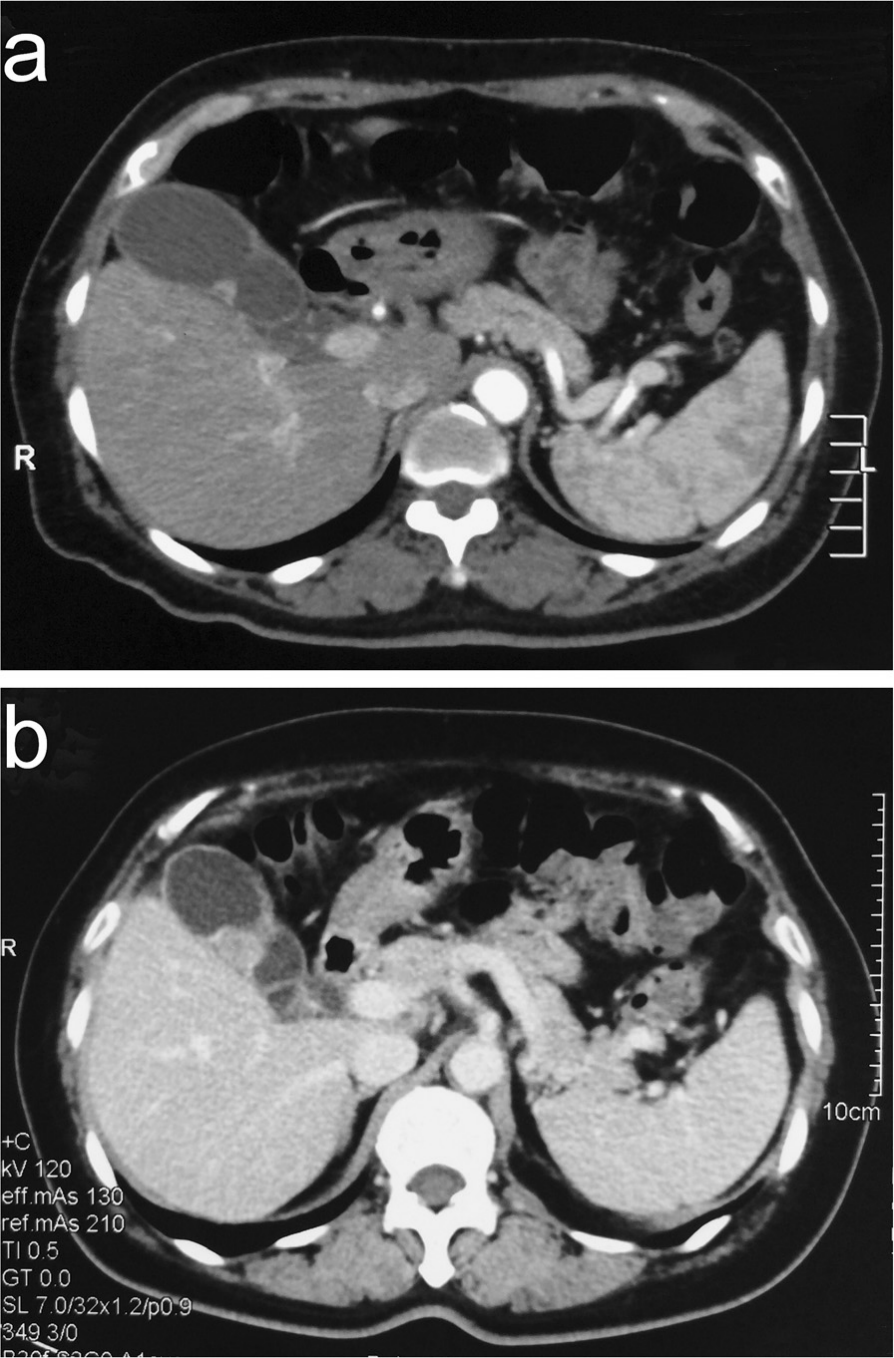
A fast-growing tumor located on the body of the gallbladder. (a) Computed tomography showed a suspected mass, measuring 0.6 cm, on the gallbladder plica 3.5 months prior. (b) At admission 3.5 months later, CT showed a 2.0 × 1.8 cm quasi-circular mass located on the body of gallbladder, with significant enhancement in the portal venous phase.
